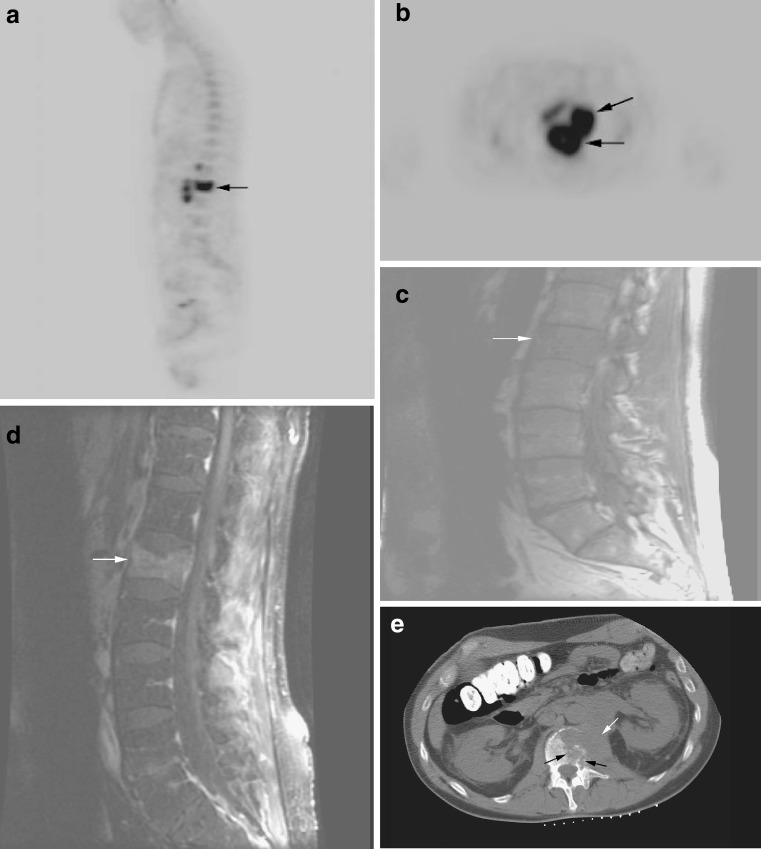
a Malignant compression fracture in a patient with lymphoma. Sagittal FDG-PET image demonstrates intense radiotracer uptake in the L1 vertebral body (SUV = 7.1;* arrow*). b Axial FDG-PET image demonstrates tumor infiltration of the L1 vertebral body with associated extra-osseous component (*arrows*). c Sagittal T1-weighted MRI demonstrates diffuse low signal intensity of the bone marrow of the lumbar spine with compression fracture of L1 (*arrow*). d Sagittal fat-suppressed T1-weighted MRI following the administration of gadolinium demonstrates diffuse enhancement of the L1 compression fracture (*arrow*). e Axial image from a CT-guided biopsy demonstrates large extra-osseous soft tissue component (*white arrow*) and infiltration of the L1 vertebral body (*black arrows*)
